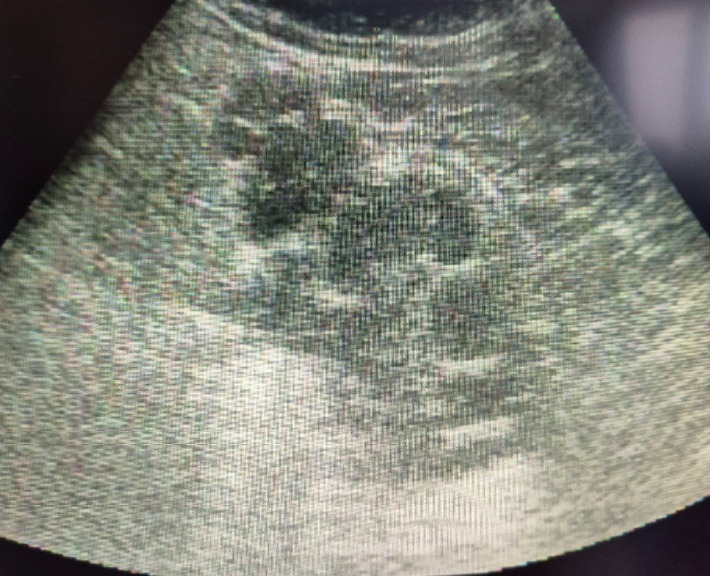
Ultrasound image of a hydatid retroperitoneal cyst with multiple scolexis.
